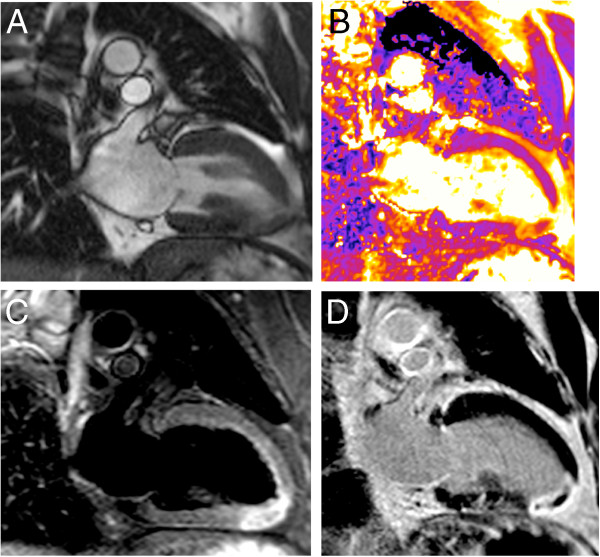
T2-mapping in acute myocardial infarction. CMR in a 47-year-old male patient 2 days after apical inferior infarction. The distal left anterior descending artery was occluded. All images shown here are in two-chamber-orientation. (A): systolic cine frame reveals apical-inferior wall motion abnormality. (B): T2-map shows an apical area of increased T2 reflected by orange color with a centre of lower T2 indicated by pink colour. (C): conventional T2-weighted STIR image shows focal area of hyperintense signal with a hypointense core. (D): Late gadolinium enhancement shows myocardial scar with core of microvascular obstruction.
